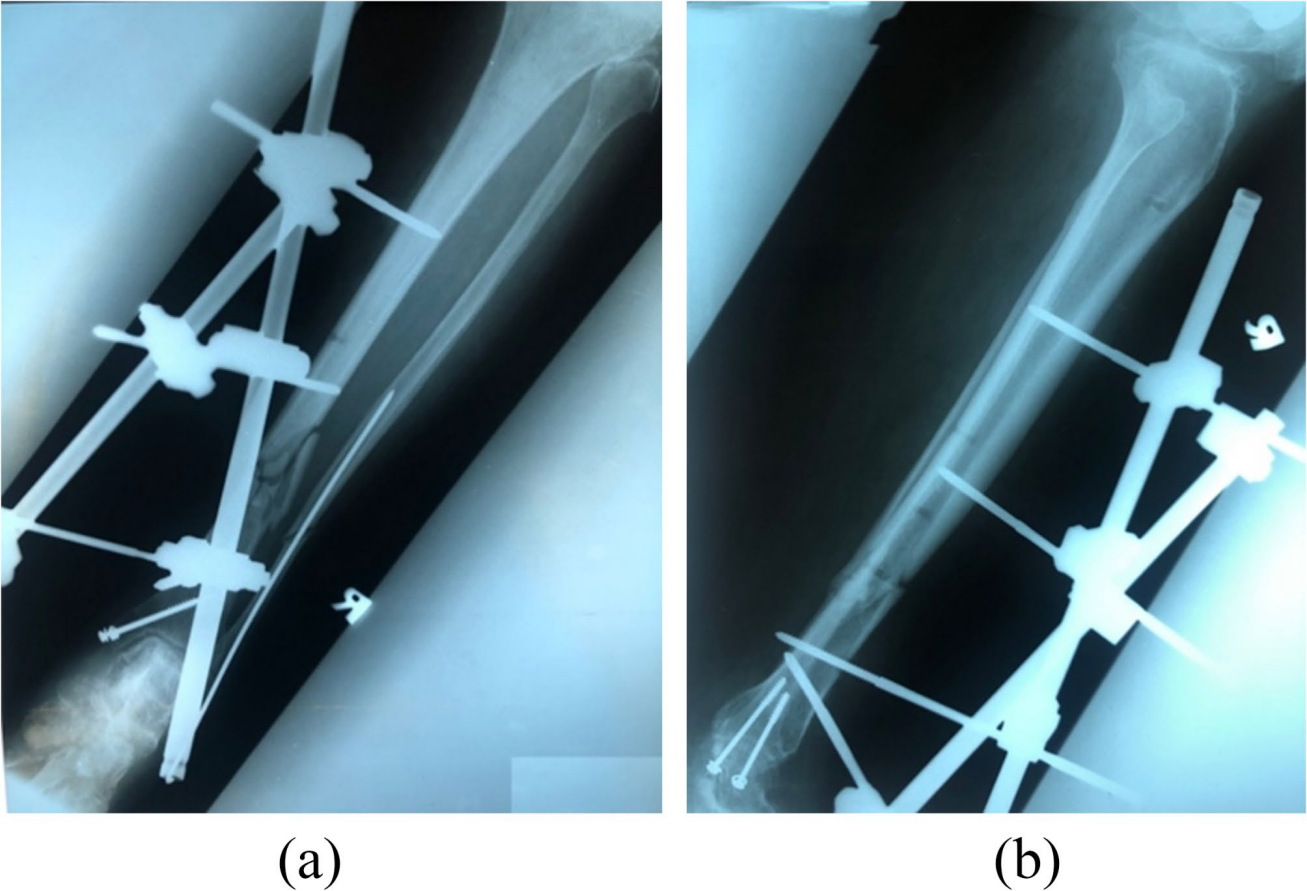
Radiographs of the locking crossed rods done on Patient A.
